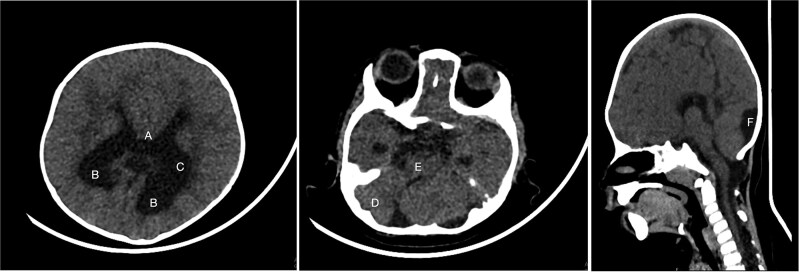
Brain CT showing: A-corpus callosum agenesis, B-colpocephaly, C-mild ventriculomegaly, D-right cerebellar and E-pontine hypoplasia, F-large cisterna magna.
